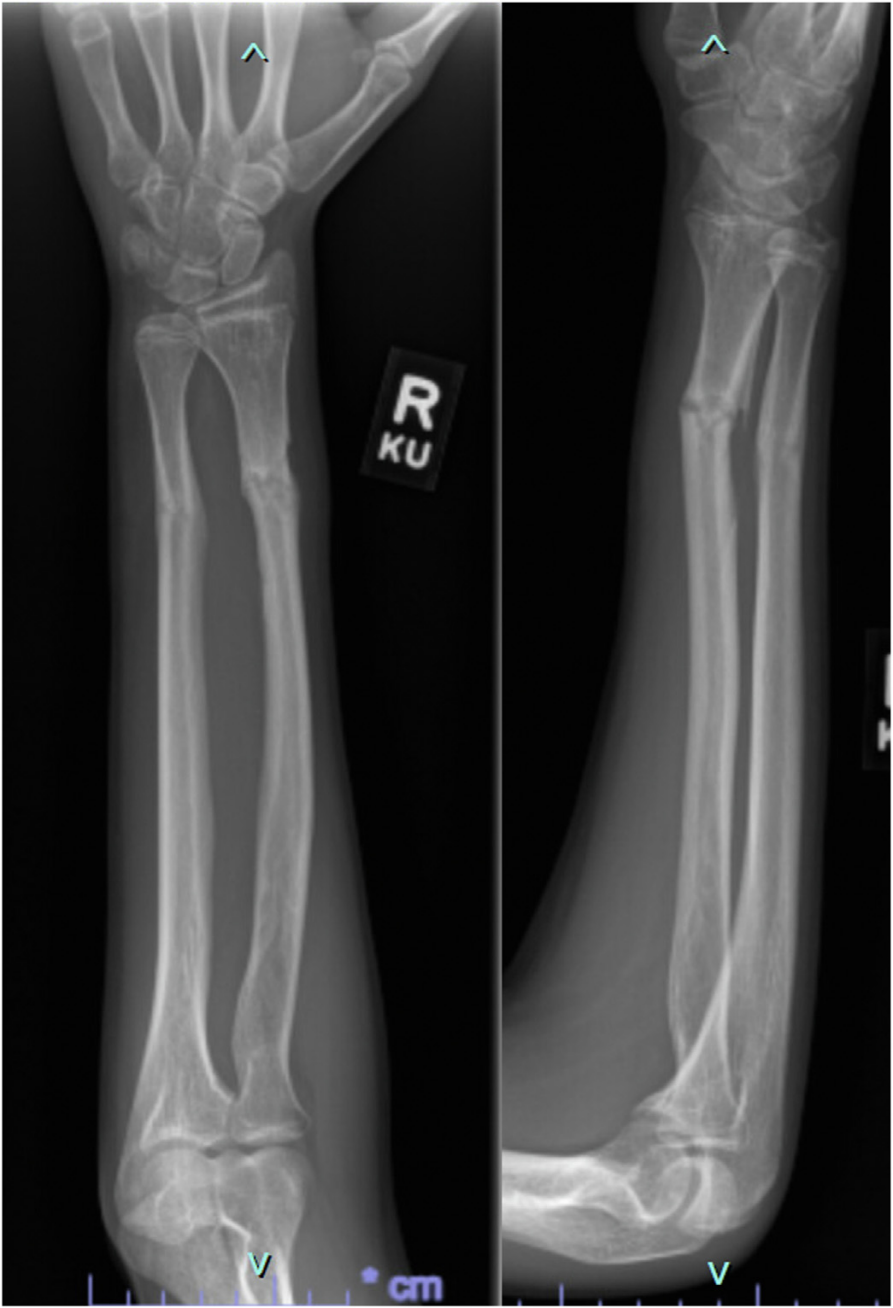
Lateral and poster–anterior radiographs taken 6 weeks after hardware removal demonstrating no evidence of abnormal fracture site lucency.
